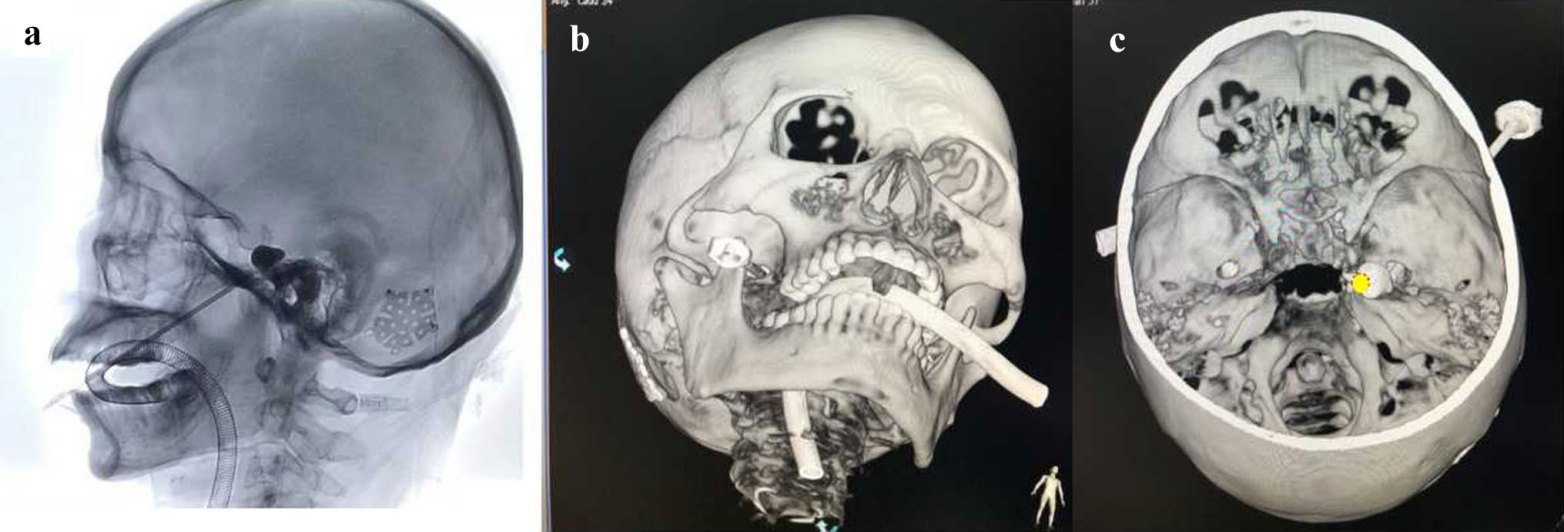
Accurate position of balloon catheter after adjustment. a Ideal pear-shaped balloon on lateral radiography. b The tip of puncture cannula is projected on the lower medial parts of the external opening of foramen ovale from external appearance of skull base in 3D reconstructed CT imaging. c The tip of balloon catheter (red dotted circle) is almost overlapped with the trigeminal porus (yellow solid dot) from internal appearance of skull base in 3D reconstructed CT imaging (color figure online)
